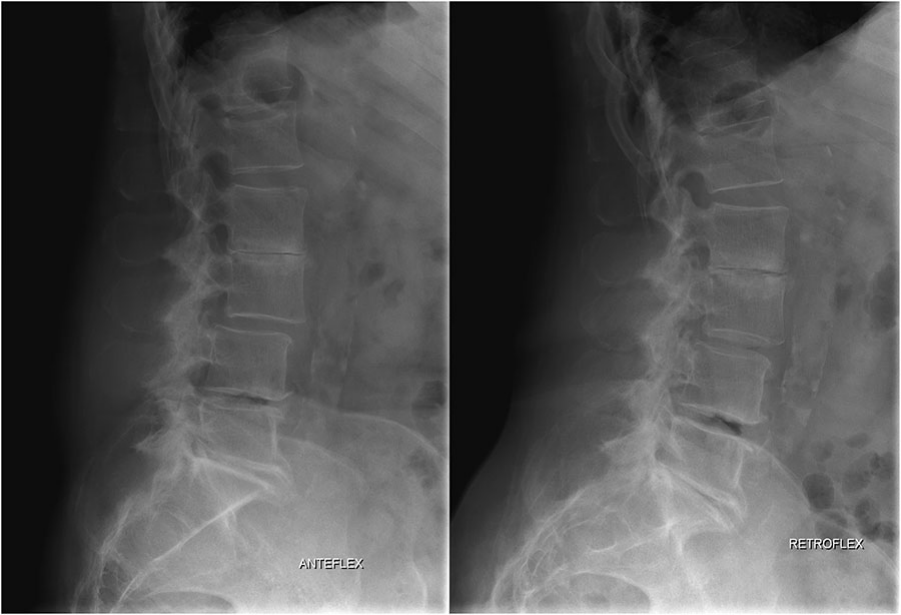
Evaluation of lumbar spine motion with dynamic x-ray prior to index surgery: flexion view (left) and extension view (right).
